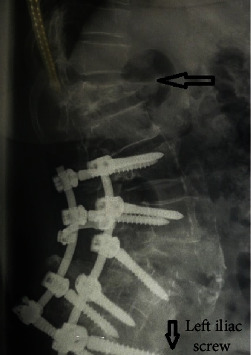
Lateral roentgenogram 18 months postoperatively showing a vertebral compression fracture of the L1 vertebra (arrow).
